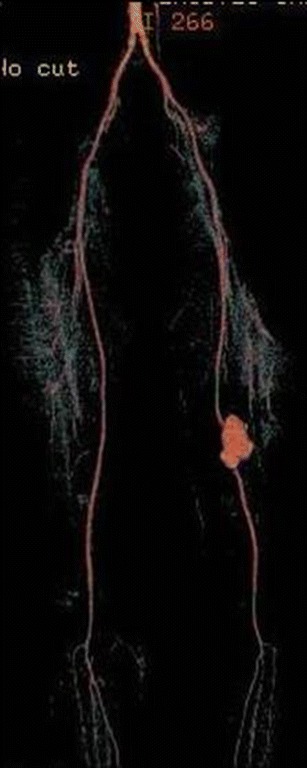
A 34-year-old male with Behçet's disease. 3D colour-coded volume-rendering CT image shows pseudoaneurysm of the superficial femoral artery
